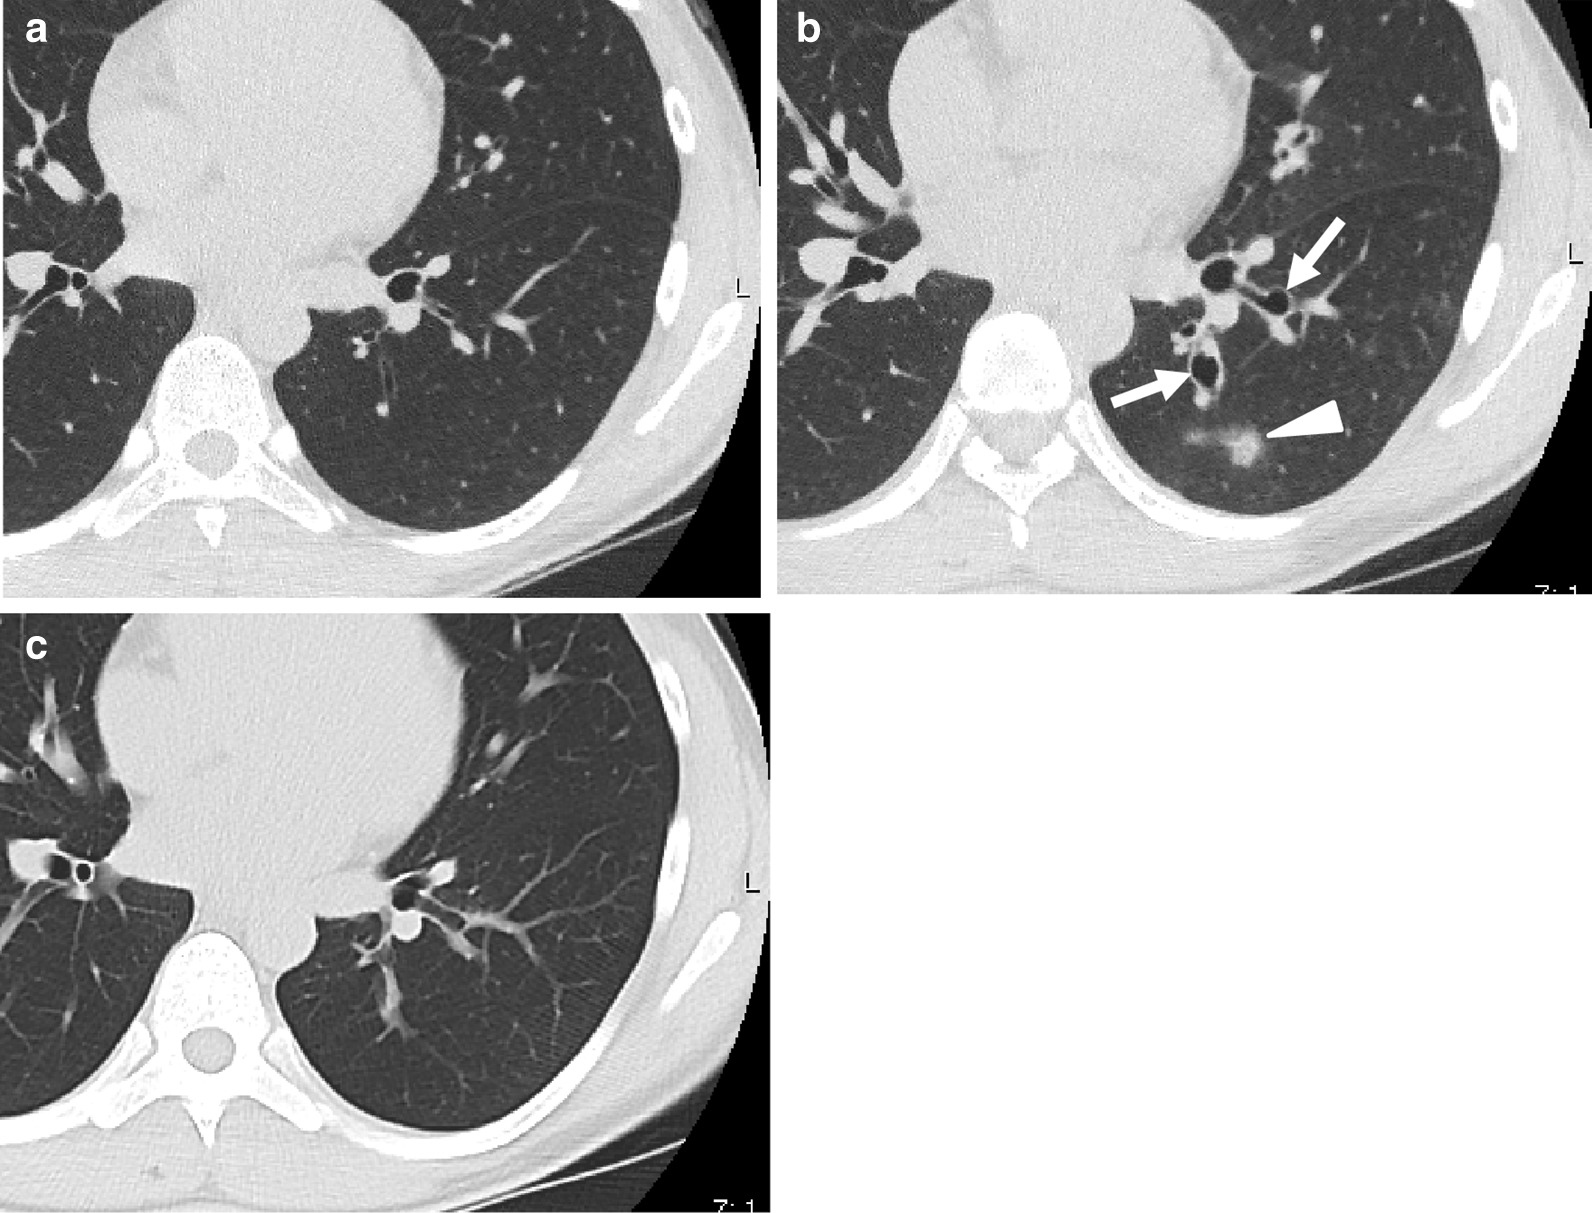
Chest computed tomography images. a Left lower lobe with thickened bronchial wall before bronchial thermoplasty (BT). b Saccular bronchodilation of left lower bronchus (B9, B10) (arrows) and nodular consolidation (triangle) are seen 3 weeks after BT. c Bronchodilation and consolidation are improved 5 months after the start of oral itraconazole administration
